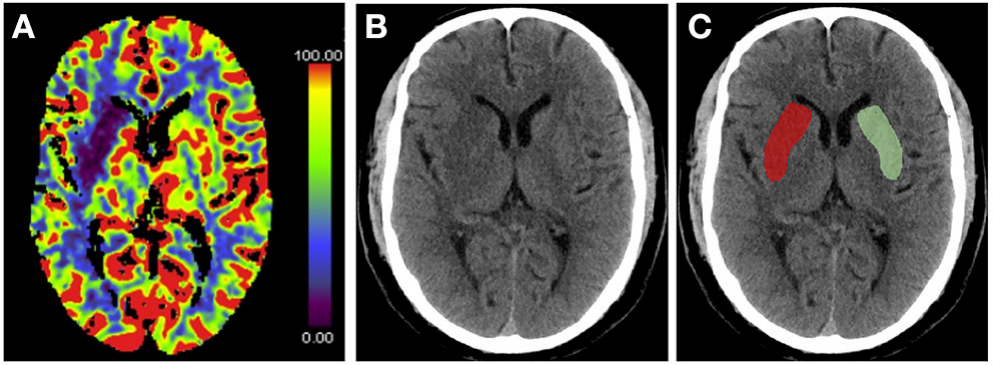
Measurement of % net water uptake (NWU) per volume on admission. (A) The initial ischemic core is identified by the initial relative cerebral blood flow (CBF) volume using a threshold of <30% in CBF maps. (B) Non-enhanced computed tomography shows a slight decrease in the density of the right basal ganglia region. (C) The mean density of the initial core (D_ischemic_) (red) was calculated in relation to the normal density (D_normal_) (green) in the homotopic contralateral region. NWU = 1 – D_Ischemic_/D_Normal_.
